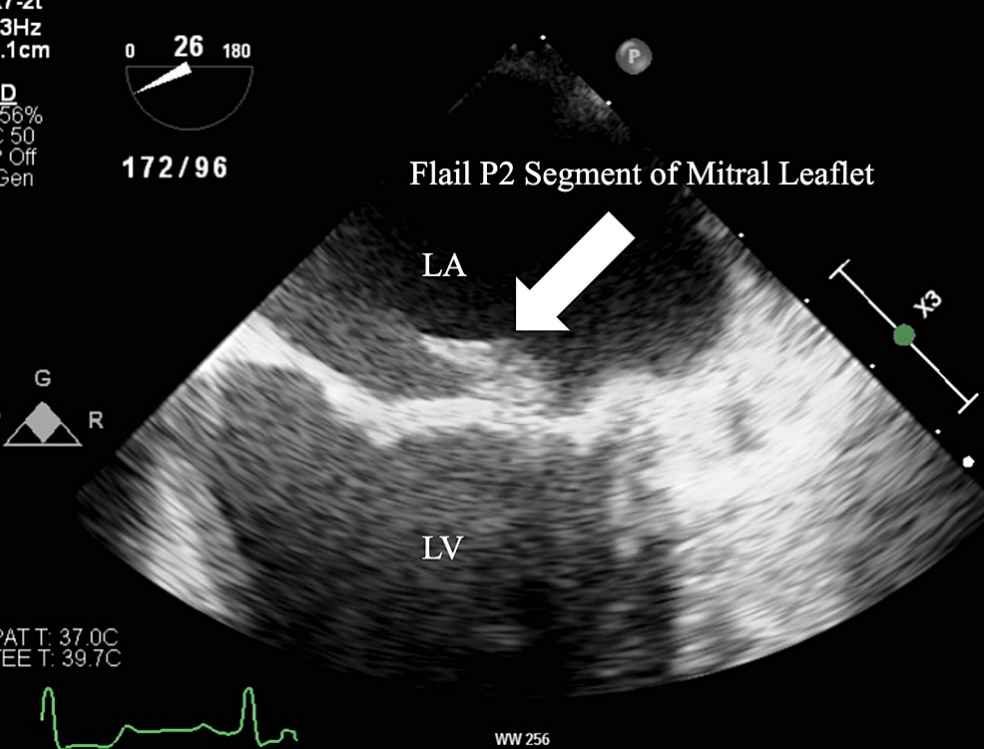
Mid-esophageal view of Transesophageal echocardiogram showing flail P2 portion of the mitral valve. LA: Left atrium, LV: Left ventricle
